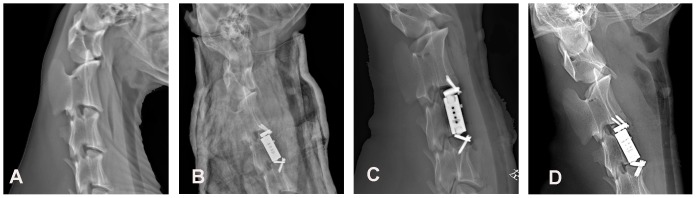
X-ray films before and after the in vivo ACVC implantation. A, Lateral X-ray film before the ACVC implantation; B, lateral X-ray film 3 weeks after the ACVC implantation; C, lateral X-ray film 6 weeks after the ACVC implantation; D, lateral X-ray film 12 weeks after the ACVC implantation.
